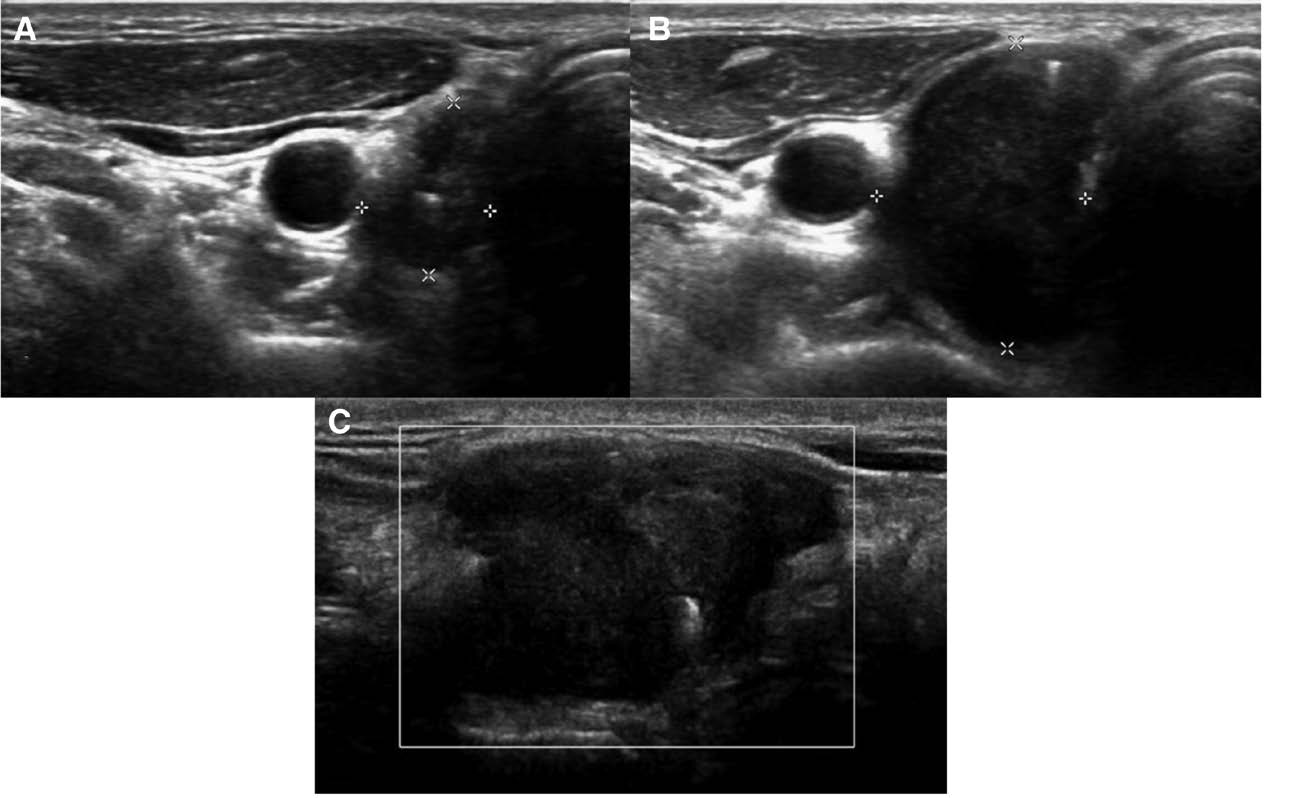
A 56-year-old male presented a thyroid surgical bed mass, detected via ultrasonography, 3 years after a total thyroidectomy for a papillary carcinoma. (A) Transverse ultrasound images revealed a 1.0 × 1.3 × 2.5 cm, irregularly-shaped, marked, hypoechoic lesion, with probable internal surgical clips. Ultrasound performed 12 months after the initial ultrasound. (B) Transverse ultrasound images showed a marked increase in the lesion size. (C) Doppler image showed no increased vascularity in the lesion.
